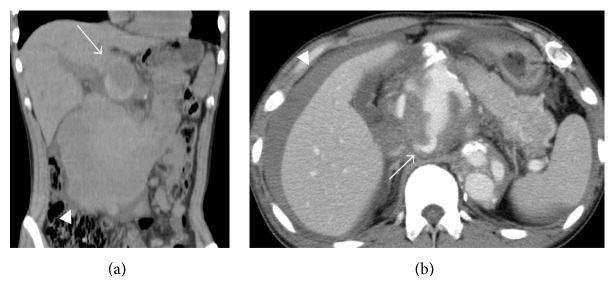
(a) Nonenhanced CT shows an aneurysm (arrow) of the common hepatic artery, with a hematoma (arrowhead) extending inferiorly. (b) Contrast-enhanced CT two and a half hours later reveals extravasation (arrow) from the aneurysm and hematoma extending to the lateral aspect of the right lobe of the liver (arrowhead).
